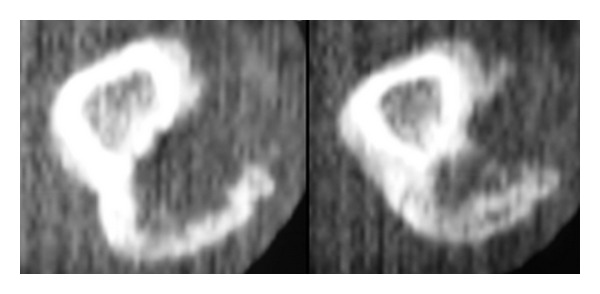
Careful axial CT scanning of the second metacarpal confirming there was not an area of continuity between the medullary cavity of the lesion and that of the underlying bone.
